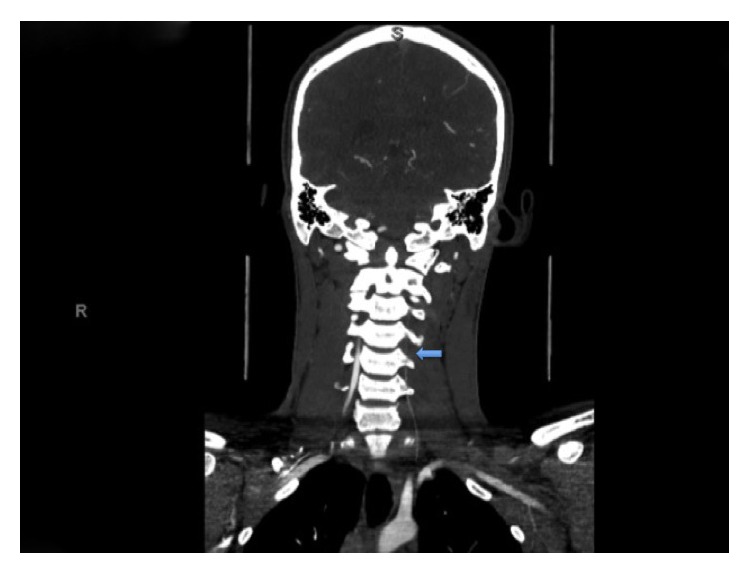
CT Angiogram shows attenuated filling of left vertebral artery (extracranial) almost in its entire length secondary to dissection (marked by solid arrow).
